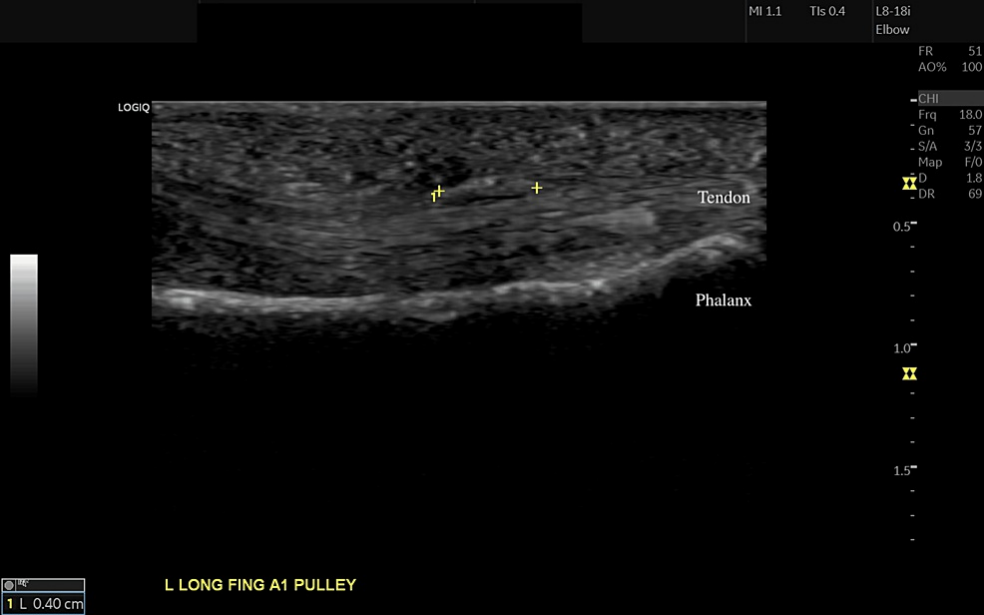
Ultrasound at 1.5 years follow up Ultrasound of the left long finger longitudinal axis. Between yellow crosses is the suture tape.
